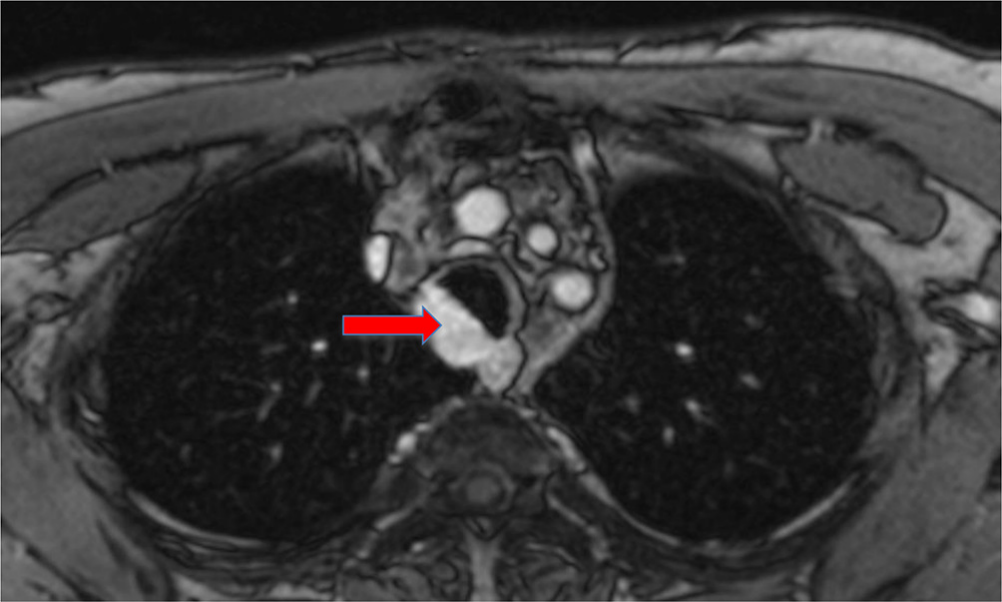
Contrast-enhanced MRI of the mediastinum: axial slice. Block arrow: recurrent tumour, pre-treatment (November 2011).
